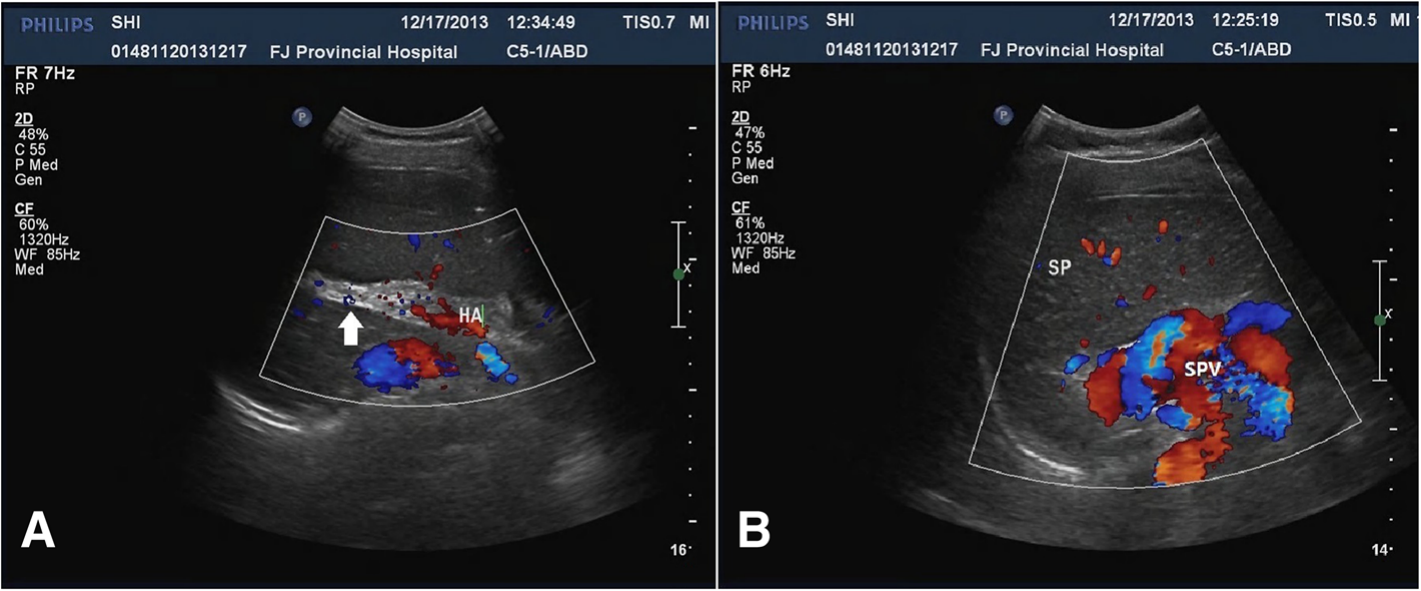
Abdominal ultrasonography shows that the intrahepatic PV system is absent (arrows) (a), and the SPV is tortuous and dilated (b). PV: portal vein; HA: hepatic artery; SP: spleen; SPV: splenic vein
